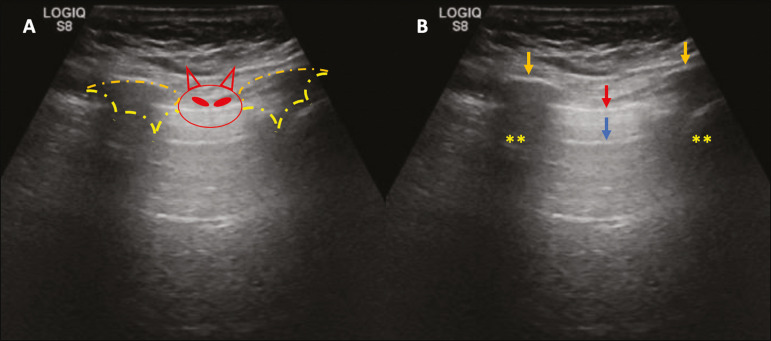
Chest ultrasound, longitudinal section in B-mode. A: “Bat sign”. B: Anatomical findings corresponding to the so-called “bat sign”. Ribs (orange arrows) and their respective acoustic shadows (asterisks). The pleura is hyperechogenic and identified between the ribs (red arrow). A-line (blue arrow) parallel to the pleura and originating from the pleural reverberation. The bat wings correspond to both the ribs and their acoustic shadows, and the bat head to the pleural echo.
